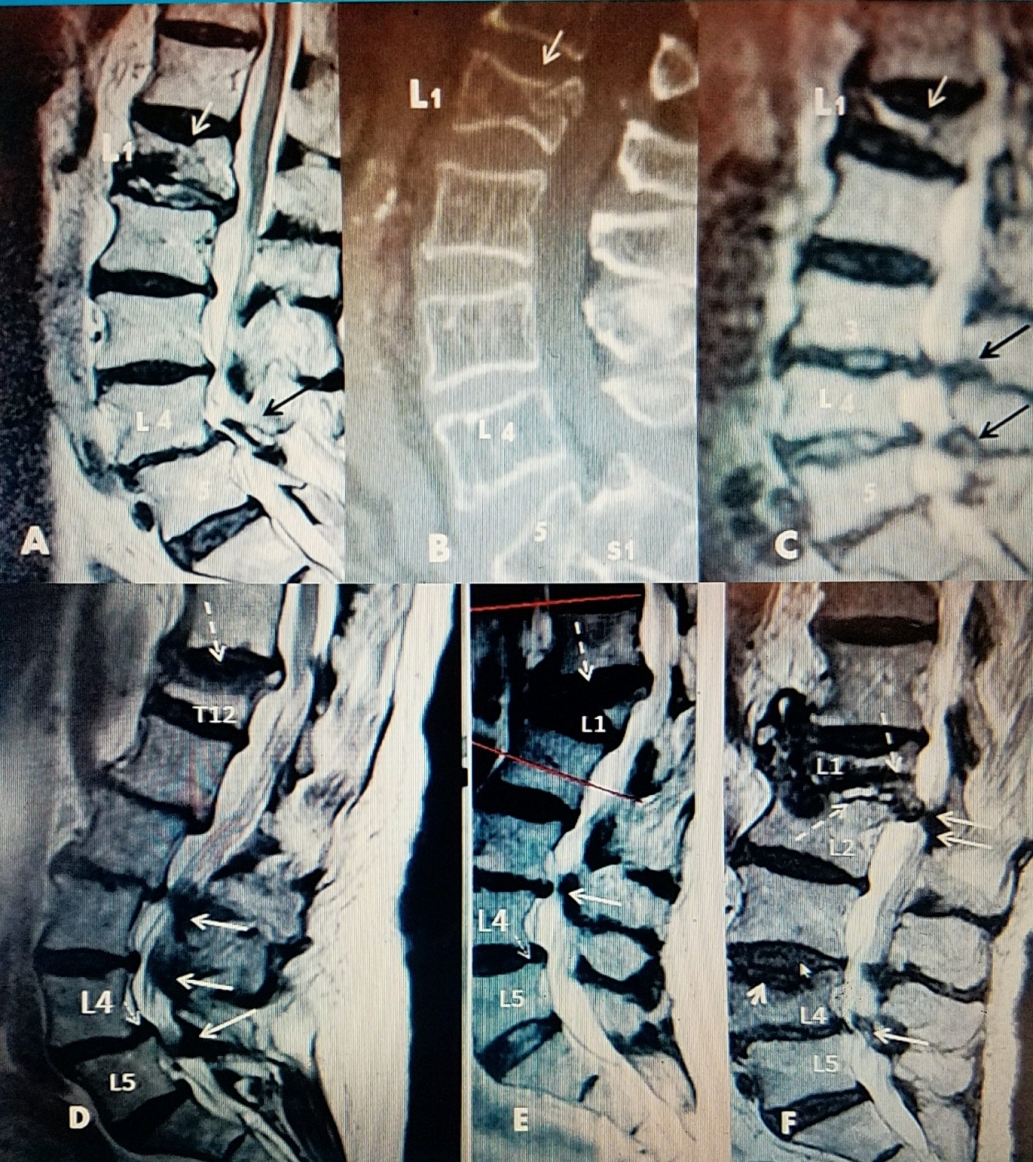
Examples of thoracic and upper lumbar fracture (fx) with lumbar spinal stenosis A: Sagittal T2 magnetic resonance imaging (MRI) scan in a 78-year-old female with preexisting L4-5 spondylolisthesis (black arrow), and new vertebral compression fracture (VCF) at L1 (white arrow) B: Sagittal computerized tomography (CT) scan of a 74-year-old female with grade 2 L5-S1 spondylolisthesis and acute L1 VCF (white arrow) C: Sagittal T2 MRI scan of a 85-year-old male with asymptomatic stenosis L3-4, L4-5 and spondylosis with acute L1 VCF (white arrow) evolving into a Vertebrae Plana D: Sagittal T2 MRI of a 72-year-old male with acute T12 70% wedge compression fracture at T12 (dotted white arrow), L2 retrolisthesis with L2-3, L3-4 and L4-5 spondylosis, and stenosis with grade 1 spondylolisthesis at L4-5 (small solid white arrow) E: Sagittal T2 MRI of a 76-year-old female with acute L1 compression fracture with a 90% collapse with kyphotic angulation (dotted white arrow). There is grade 1 anterolisthesis at L4-5 (thin white arrow). There is L3-4 spondylosis with sagittal stenosis (solid white arrow). F: Sagittal T2 MRI of a 71-year-old female with post kyphoplasty collapse at L1 (dotted white arrow) with posterior canal compression (2 white arrows) and early edema of anterior superior endplate of L2. A new edematous fracture of superior endplate of L4 (small solid white arrow) with posterior stenosis at L4-5 (single solid white arrow).
